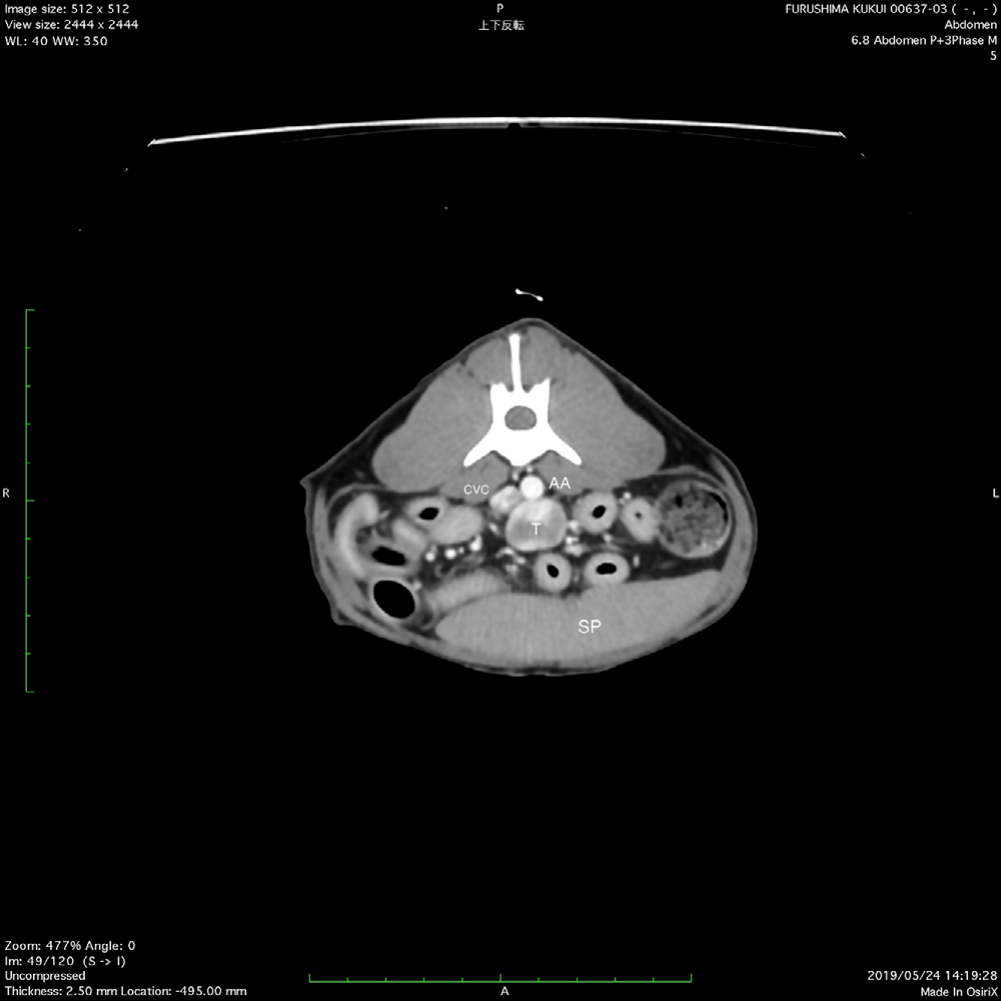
Preoperative computed tomography image. The retroperitoneal tumour was located close to the CVC and AA. AA: abdominal aorta; CVC: caudal vena cava; SP: spleen; T: tumour
